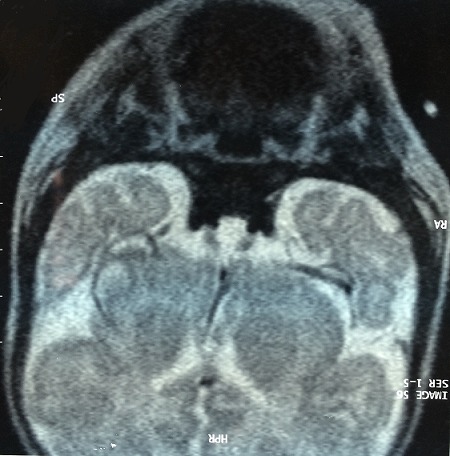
MRI of total empty sella: Coronal weighted T1 image showing arachnoidocele intra-sellar
